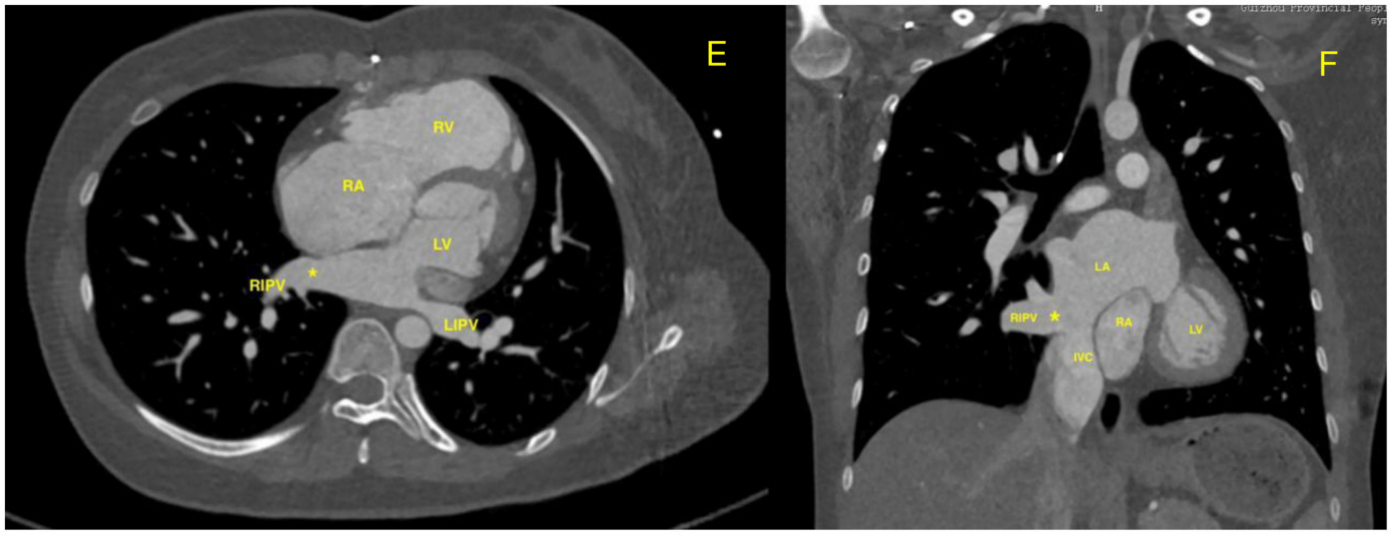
(E,F) Contrast-enhanced CT (cross-sectional and coronal views) showing the abnormal communication(*) between the right lower pulmonary vein and the inferior vena cava. The inferior vena cava drains normally into the right atrium; the apparent proximity to the left atrium is due to imaging overlap.
